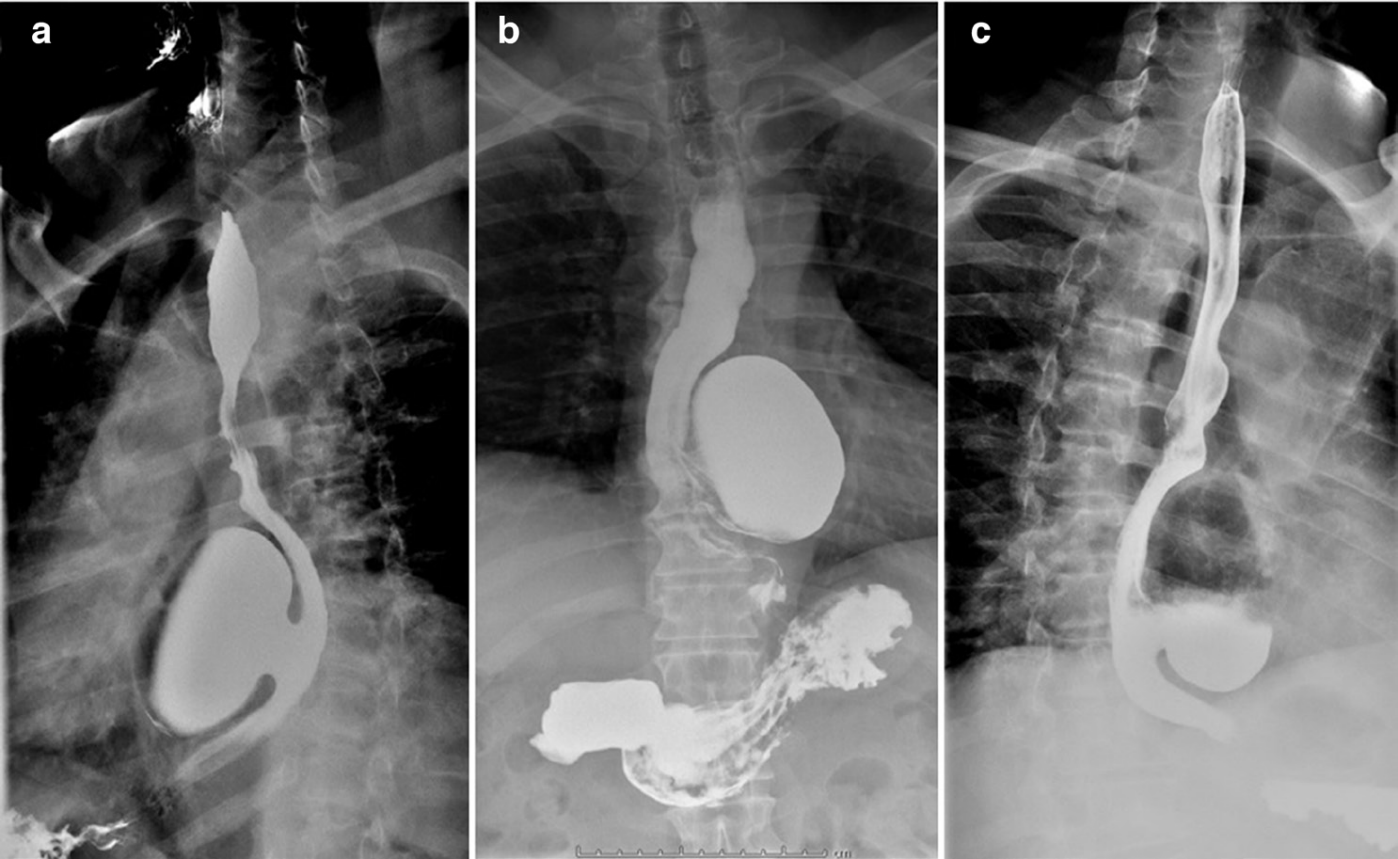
Barium swallowing X-ray showed the large epiphrenic esophageal diverticulum on the left anterior wall with 2,5 cm neck, in the right oblique projection (A), AP projection (B) and left oblique projection (C). AP, anteroposterior.
